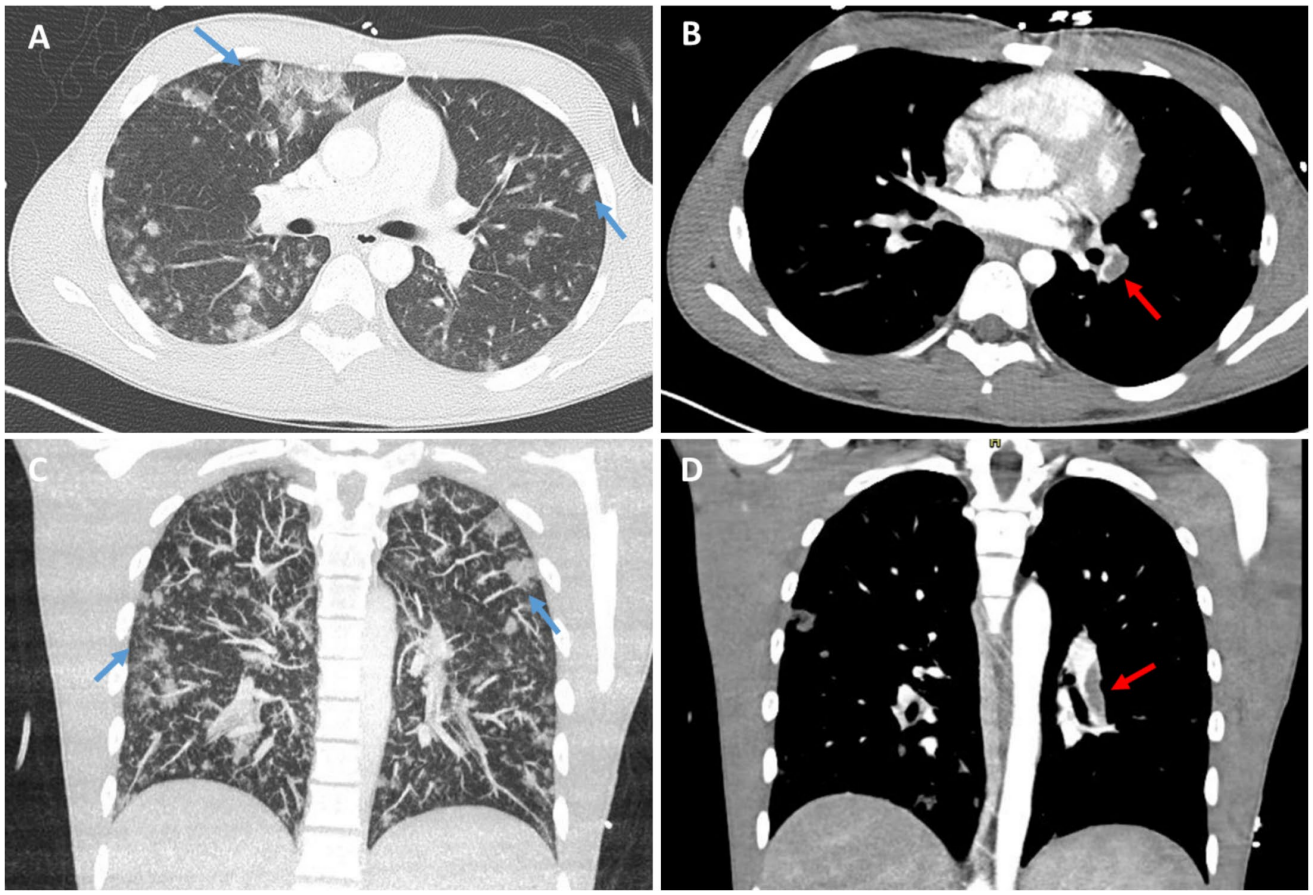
Chest CT findings in a 16-year-old male with PVL-positive *Staphylococcus aureus* sepsis. (A,C) Axial (A) and coronal (C) lung window images show multifocal bilateral peripheral ground-glass opacities and consolidations (blue arrows), suggestive of acute inflammatory or infectious process, compatible with septic pulmonary emboli or hemorrhagic pneumonia. (B,D) Axial (B) and coronal (D) contrast-enhanced mediastinal window images demonstrate filling defects within the right pulmonary artery (red arrows), suggestive of acute pulmonary embolism.
